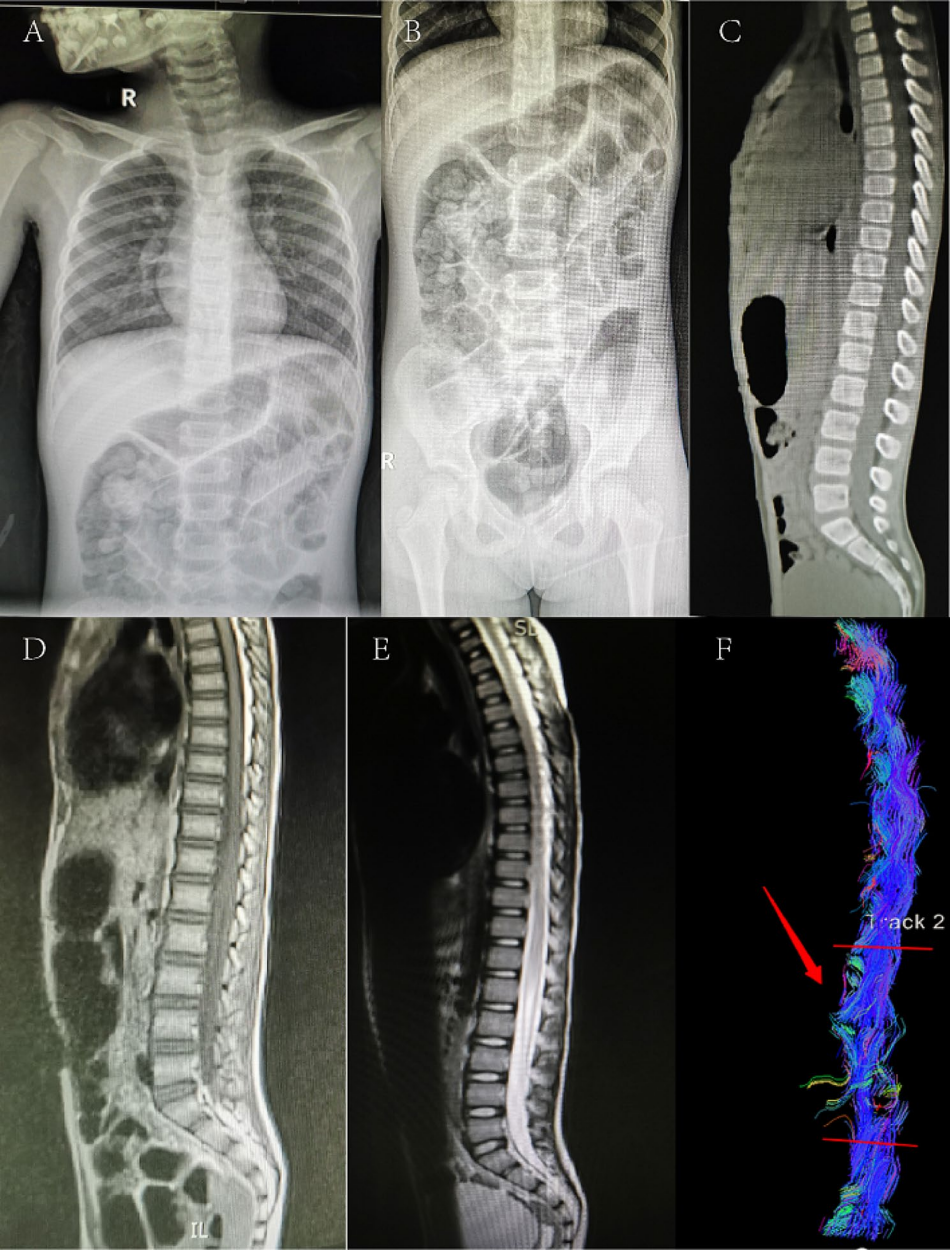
An 8-year-old female patient presented with paralysis after dance backbend practice. X-ray (A, B) and CT (C) scans did not detect any fractures or dislocations. MRI (D, E) examination also showed no apparent abnormalities. However, DTI fiber reconstruction images (F) revealed localized thinning, fracture, and sparse fibers below the ninth thoracic vertebra
